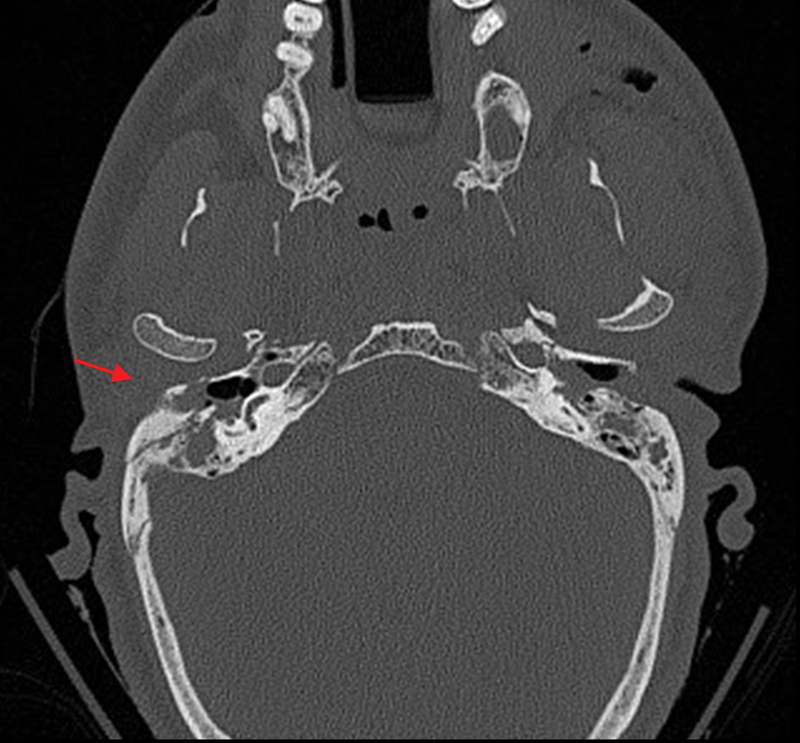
Computed tomography shows mastoid fracture with extension to the external auditory canal (arrow).
